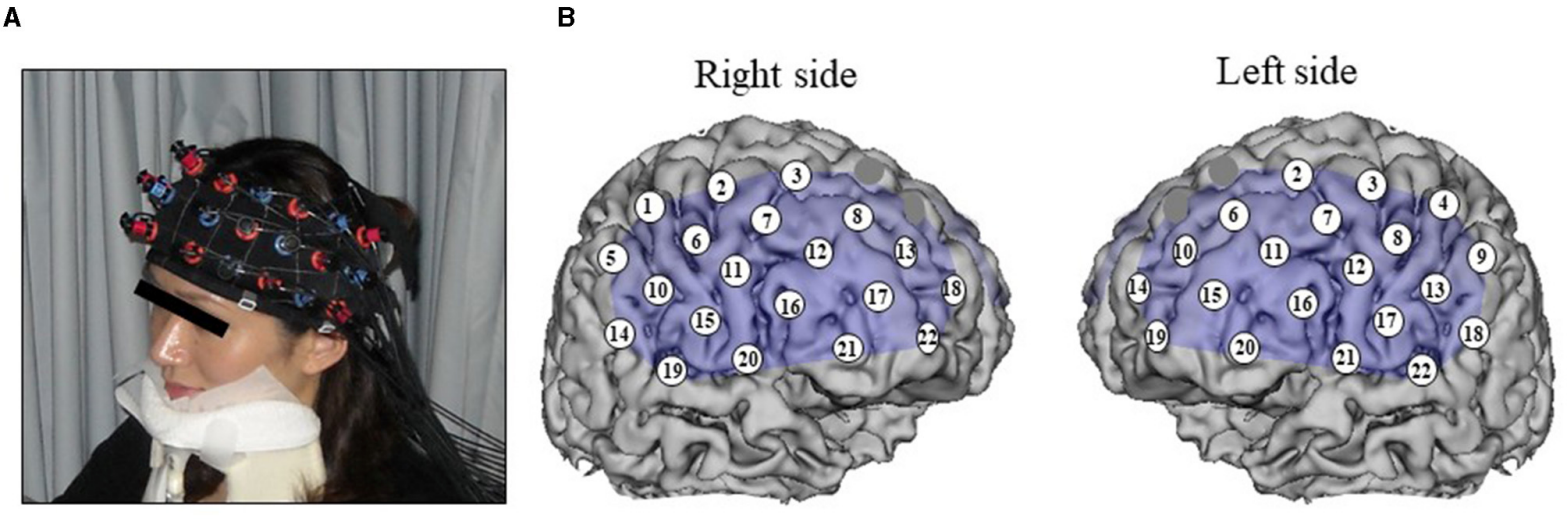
Measurement point of 44 channels for NIRS. (A) Jaw fixation in order to eliminate influence of head movement. (B) Spatial position of the channels arranged of magnetic resonance imaging.
